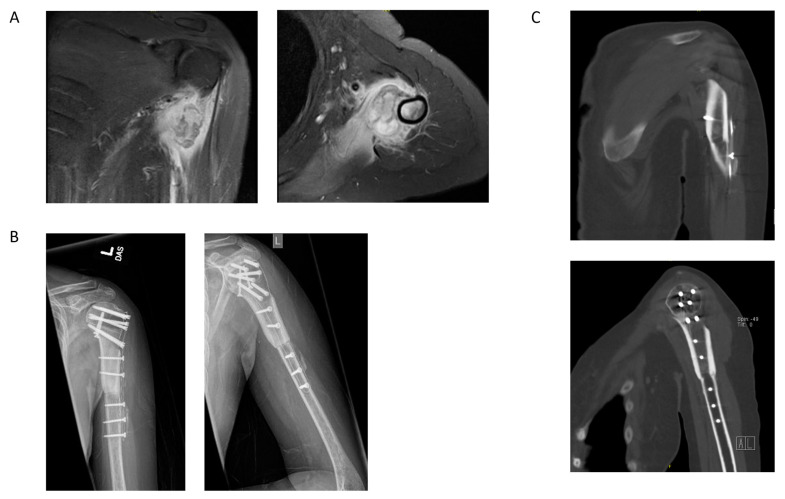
(A) Pre-operative T1 post-contrast, fat-saturation coronal and axial magnetic resonance (MR) images showing a large, enhancing tissue mass on the left proximal humerus without marrow involvement. (B) The 1-month post-operative AP and lateral radiographs following left humerus intercalary resection and allograft reconstruction with carbon fiber plate and cortical screws demonstrating incomplete fusion between the allograft and the native humerus. (C) The 8-month post-operative coronal and sagittal computed tomography (CT) images showing partial fusion. The carbon fiber plate facilitated visualization of the site of allograft and native humerus fusion status.
